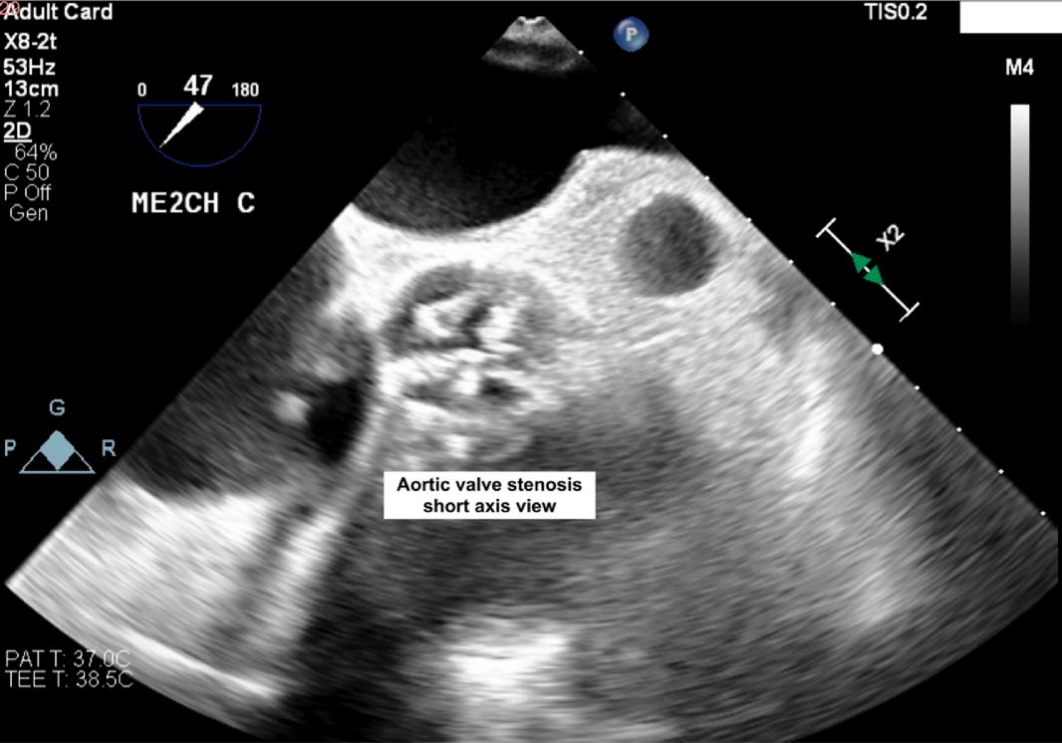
Transoesophageal echocardiography in short axis view without colour Doppler demonstrating the presence of a highly calcific and stenotic aortic valve.
